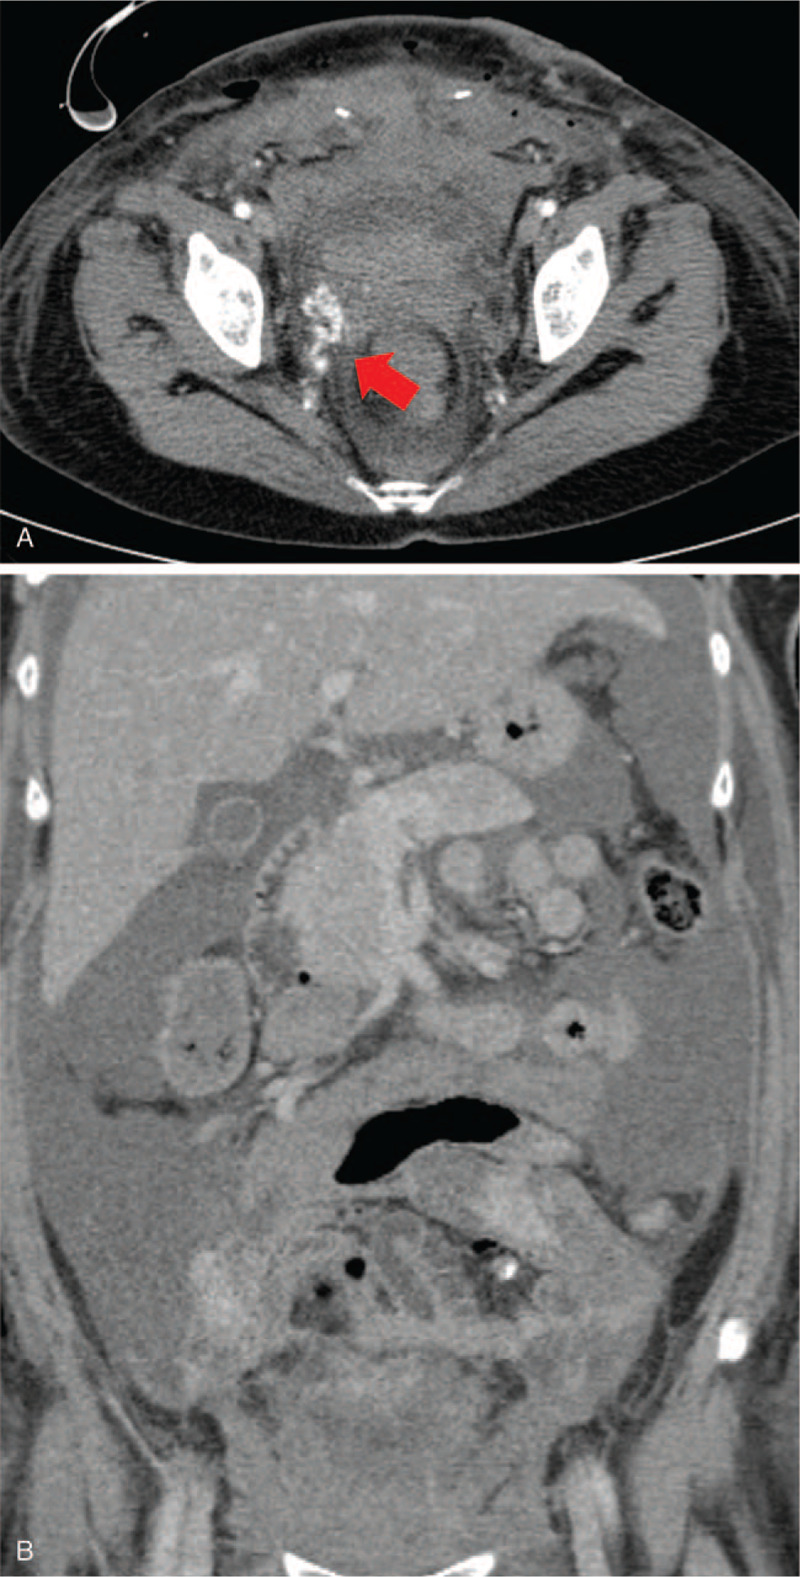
A 34-year-old woman (gravida 2, para 2) transferred to our hospital for persistent vaginal bleeding after cesarean section. The computed tomography (CT) scan 2 days after hysterectomy shows abnormal tortuous hypertrophied vascular structure at right lateral aspect of the pelvic cavity (red arrow) on axial image (A) and a large amount of hemoperitoneum on coronal image (B).
